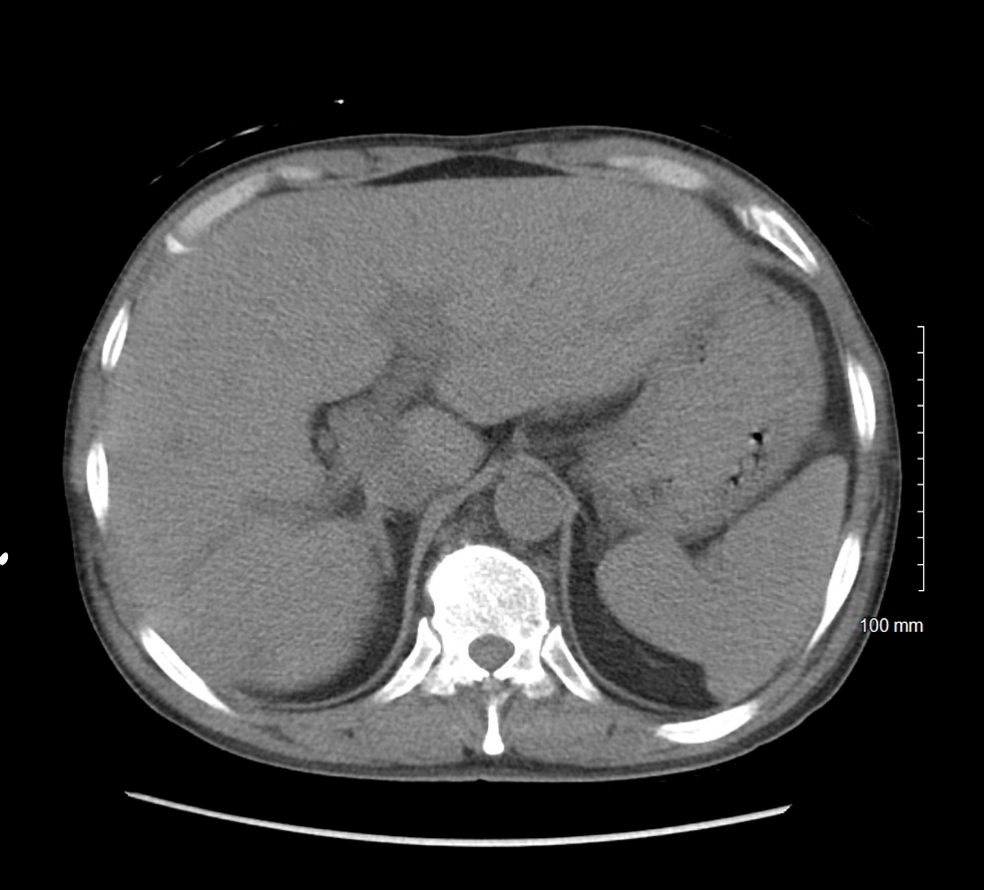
CT scan of the abdomen in the patient with acute disseminated histoplasmosis with no evidence of Liver or Spleen lesions.
